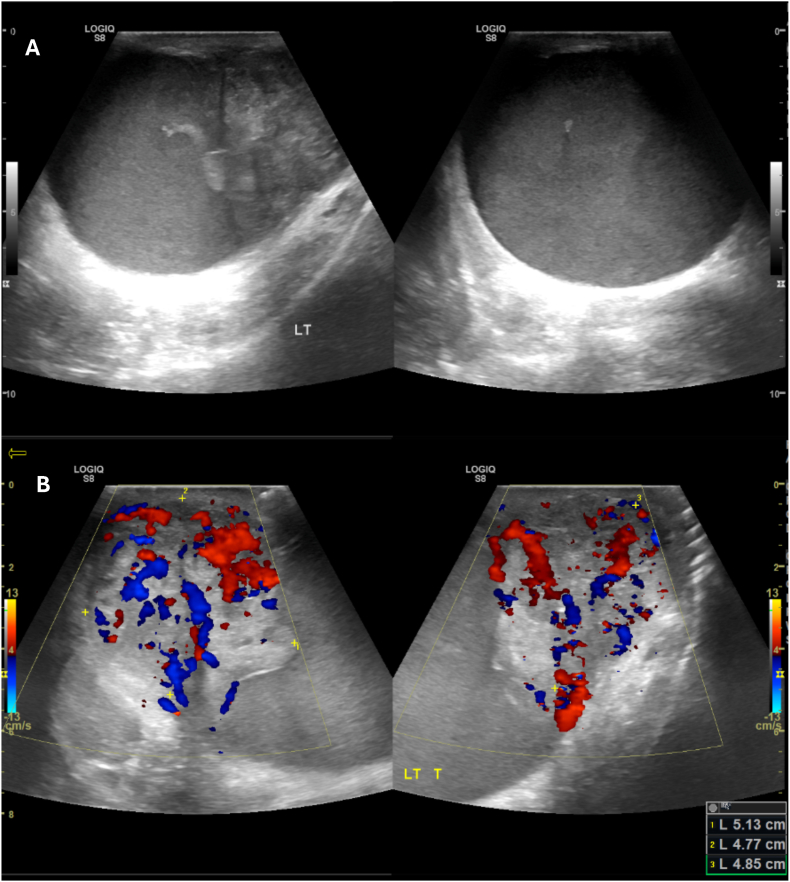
Ultrasound Testes. Panel 1A: Ultrasound of left testes showing solid tumour and lack of normal testicular architecture. Panel 2A: Ultrasound of left testes with blood flow overlay showing increased blood flow.
